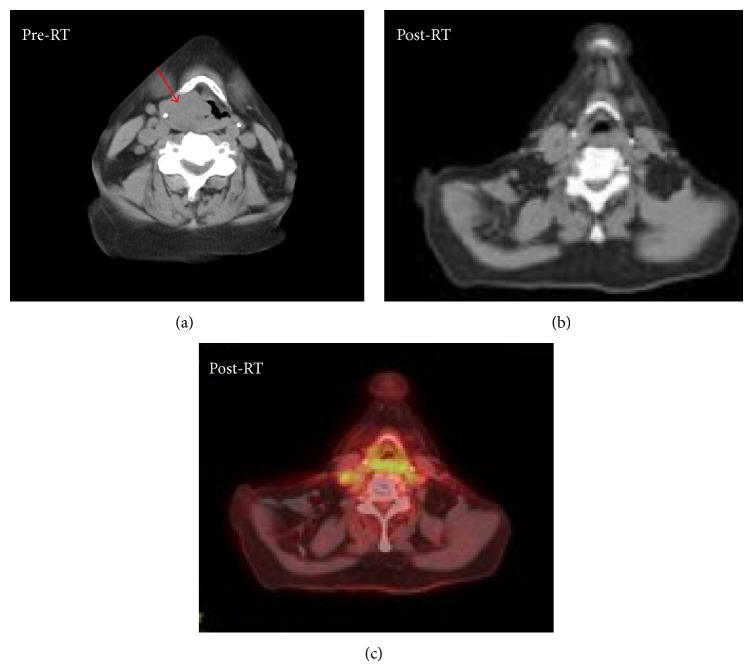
Complete response following radiation therapy. The mass (red arrow) present on CT-based imaging performed at diagnosis (a) has resolved 3 months following radiation (b) and there is no corresponding PET avidity (c).
